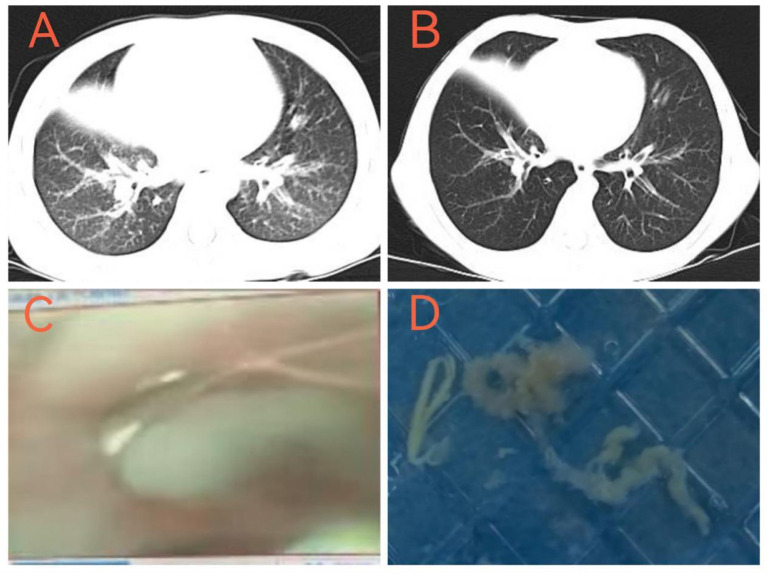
(A), Chest CT scan on the day after admission, revealing atelectasis of the right middle lobe; (B), Follow-up chest CT scan on day 9 of hospitalization, showing persistent atelectasis of the right middle lobe; (C), Bronchoscopic image demonstrating the right middle lobe bronchus obstructed by white gelatinous material; (D), Image showing the cast embolus extracted from the right middle lobe bronchus.
